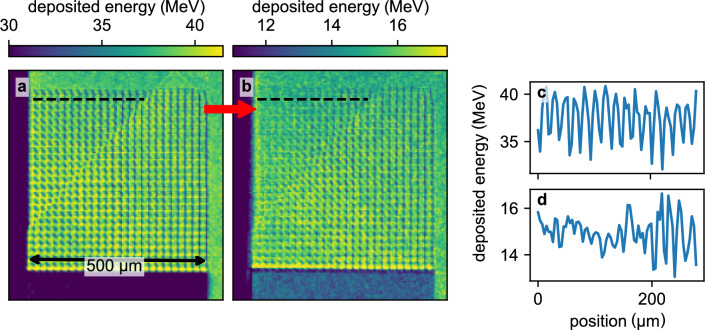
Homogenization of 3D printed foam structure after a delay of $$300\,\text {ps}$$. (a) shows the measured intensity of the cold sample as deposited X-ray energy per pixel, (b) shows the same sample after heating by protons. The red arrow indicates the position of the incident protons. The angled line in both images is caused by a small, non-sensitive gap in the tiled detector. The pixel values of the pixel rows indicated by the black dashed line are presented again in (c) and (d) for the cold and heated sample respectively.
